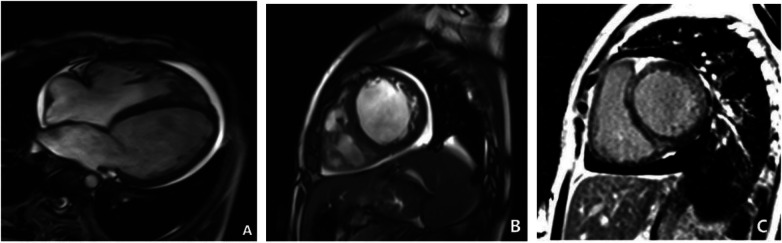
Cardiac MRI on September 23, 2021. (A,B) Show short-axis and four-chamber cine images that suggest left ventricular enlargement, reduced wall motion, and increased free wall thickness. (C) Showed delayed enhancement imaging in the short-axis view, which revealed linear enhancement between the mid-septal myocardium and the mid-lateral left ventricular wall.
